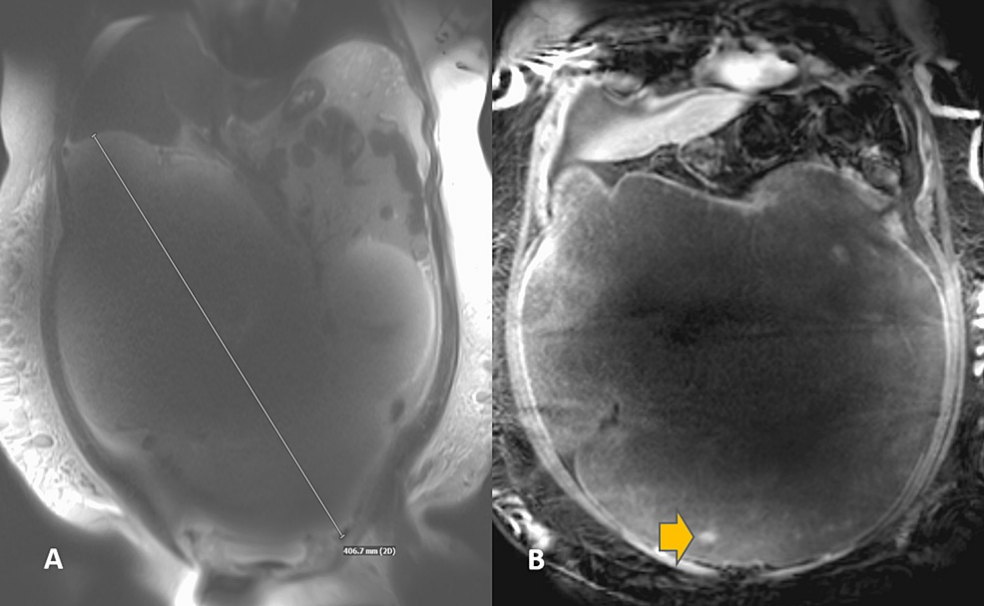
Coronal T2-weighted MR image A: Coronal T2-weighted image showing a giant infra-mesocolic abdominal mass measuring close to 40 cm. B: Coronal post-contrast image showing areas of solid enhancement (arrow) within the mass.
